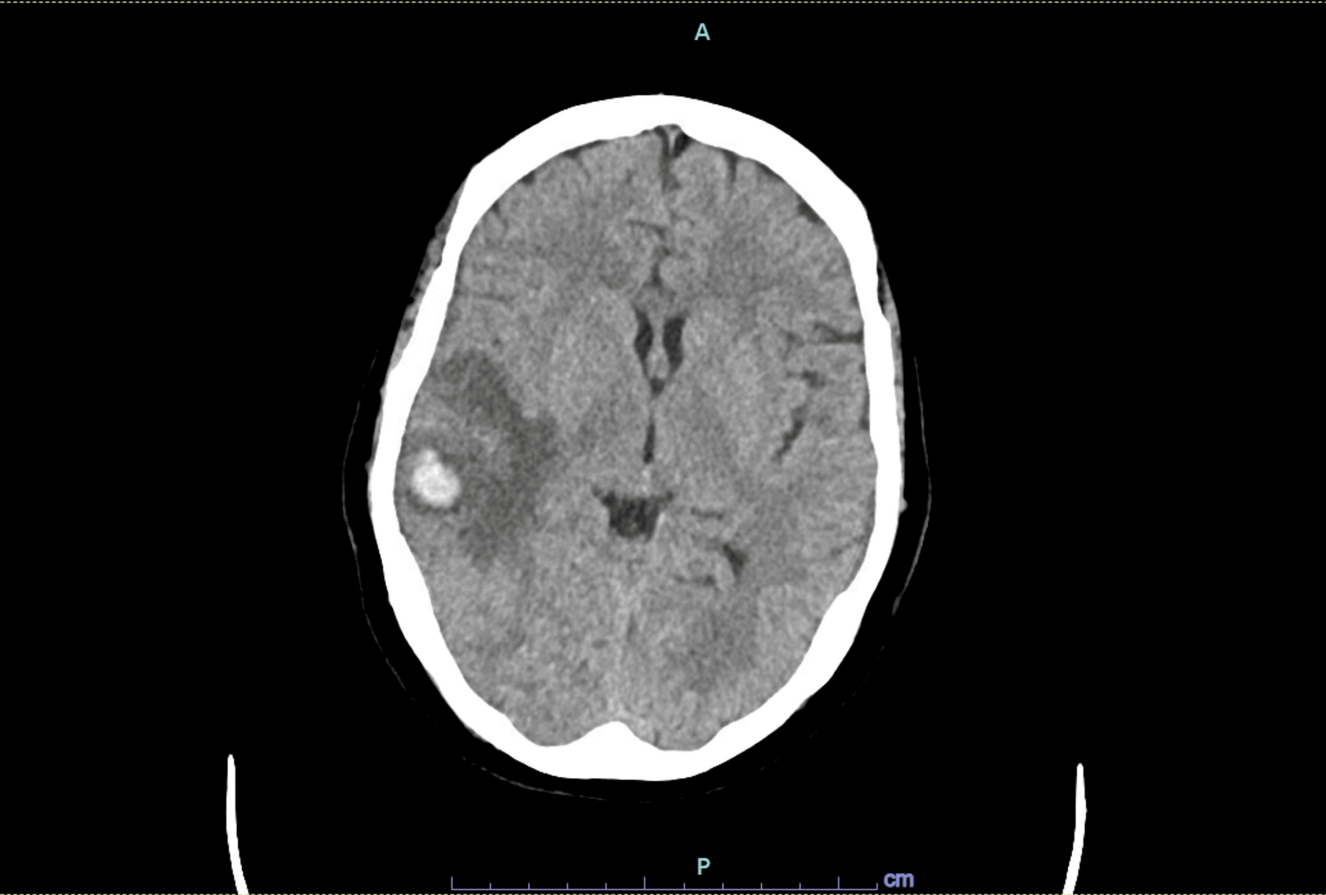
CT head without contrast showing acute right temporal intraparenchymal hematoma (axial view)
